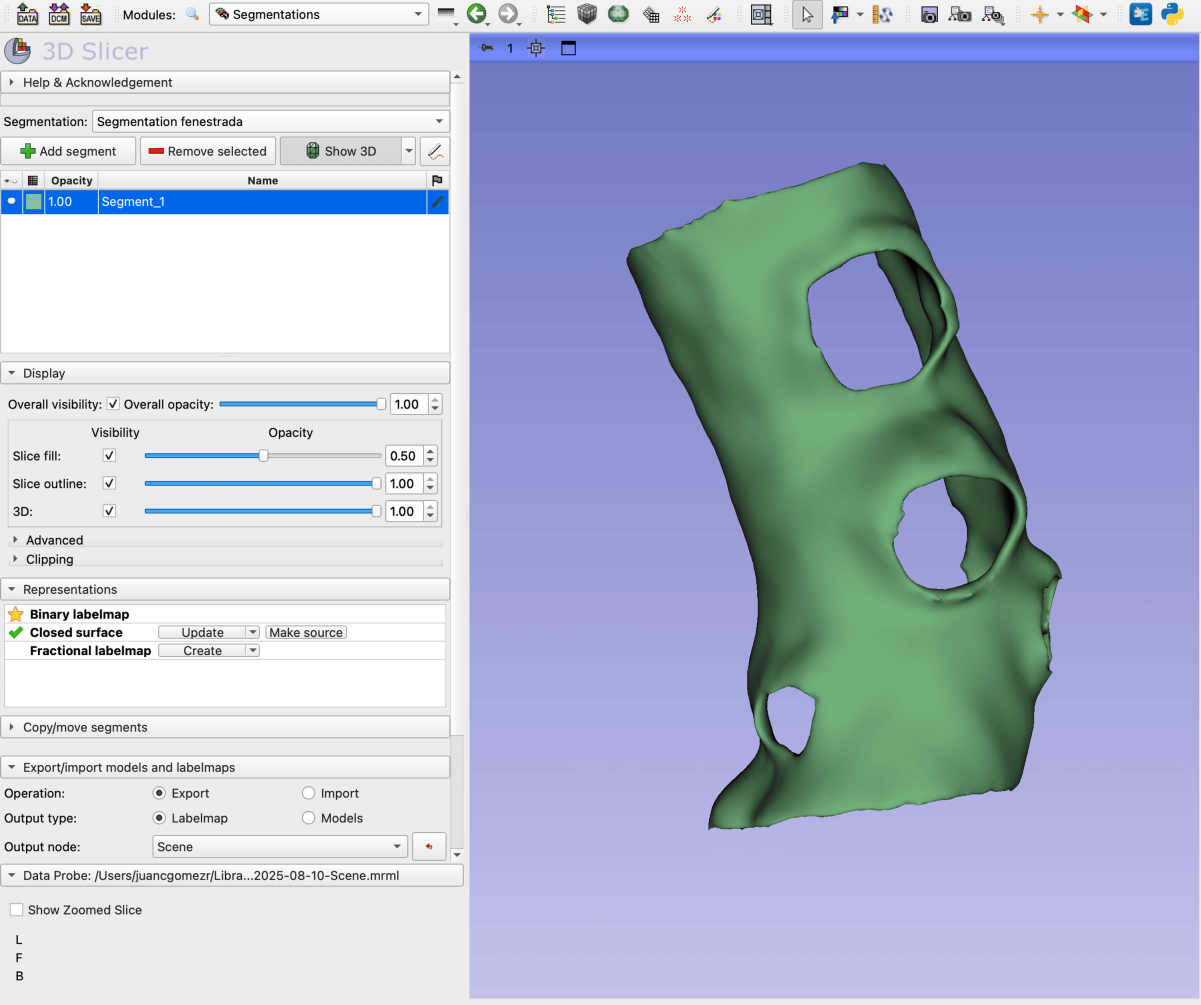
Screenshot of the 3D Slicer Segmentation module showing the fenestrated aortic model Screenshot from 3D Slicer displaying the Segmentation module interface. The left panel shows the control menus, including Display, Representations, and Copy/Import segment options. On the right, the 3D view presents the hollowed aortic model generated from the Computed Tomography Angiography (CTA) Digital Imaging and Communications in Medicine (DICOM) dataset using the Grow from Seeds segmentation and further processed with the Hollow tool. A precision cut was made with the Scissors tool at the level of the visceral ostia, creating the open-ended appearance of the celiac trunk, superior mesenteric artery, and renal arteries.
